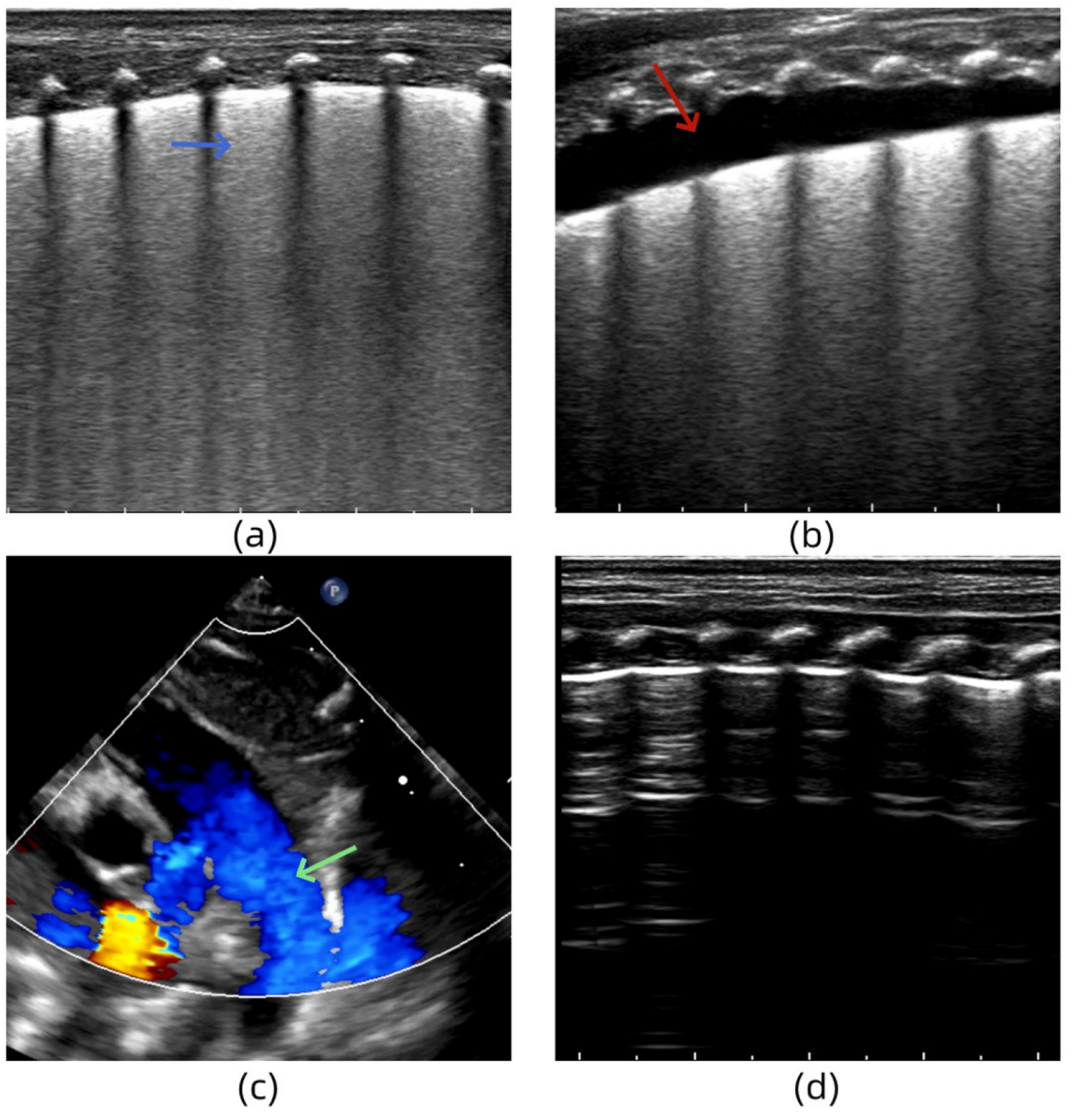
(a) Lung ultrasonography (LUS) reveals the dense B-lines in both lung fields. (b) LUS shows a small amount of pleural effusion in the lungs. (c) Echocardiography reveals a right-to-left shunt of the ductus arteriosus. (d) LUS findings return to normal.
